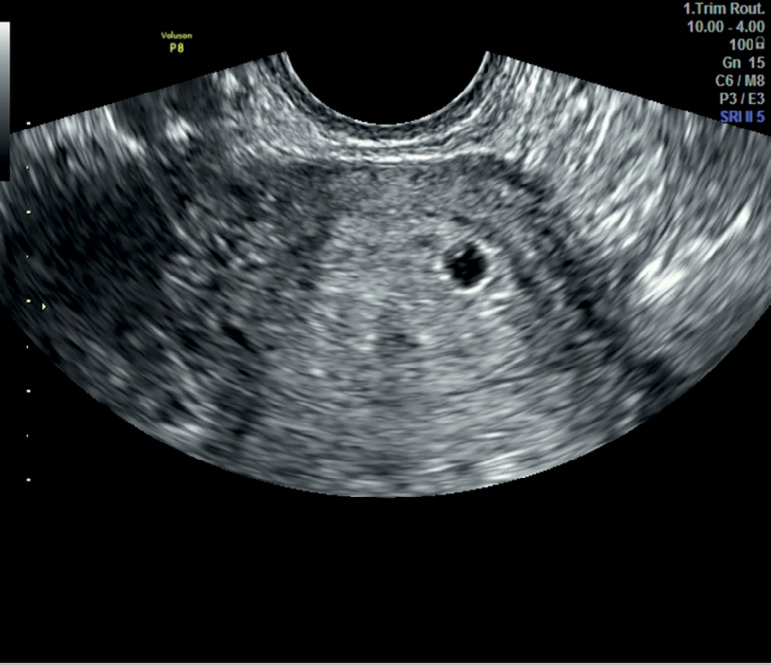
Ultrasound with early gestation sac.
